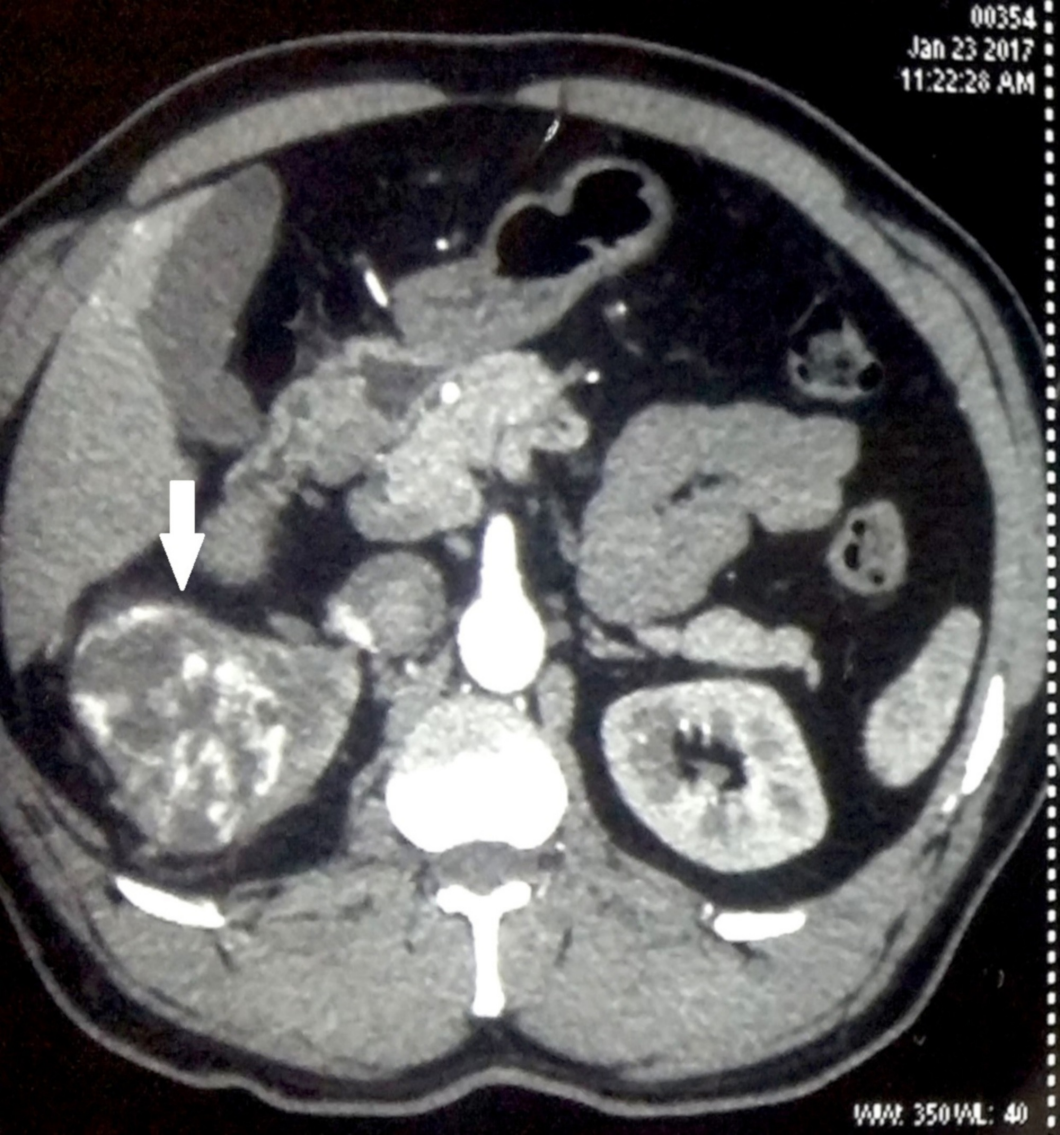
Contrast-enhanced computed tomography (CT) of the abdomen showing an enhancing heterogeneous tumor occupying the right kidney (white arrow)
